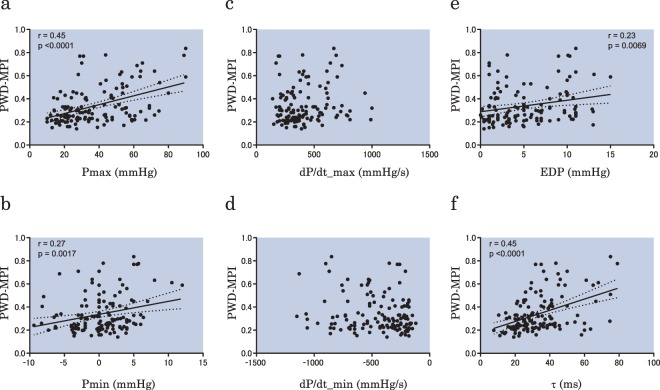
Correlations between PMD-MPI and RV pressure parameters obtained from cardiac catheterization. There are significant correlations between PWD-MPI and Pmax (a), Pmin (b), EDP (e), and τ (f), whereas there are no significant correlations with the dP/dt_max (c) and dP/dt_min (d). PWD-MPI, myocardial performance index evaluated using pulsed-wave Doppler; Pmax, maximum right ventricular pressure; Pmin, minimum right ventricular pressure; dP/dt_max, maximum time derivative of right ventricular pressure; dP/dt_min, minimum time derivative of right ventricular pressure; EDP, right ventricular end-diastolic pressure; τ, time constant of right ventricular relaxation.
